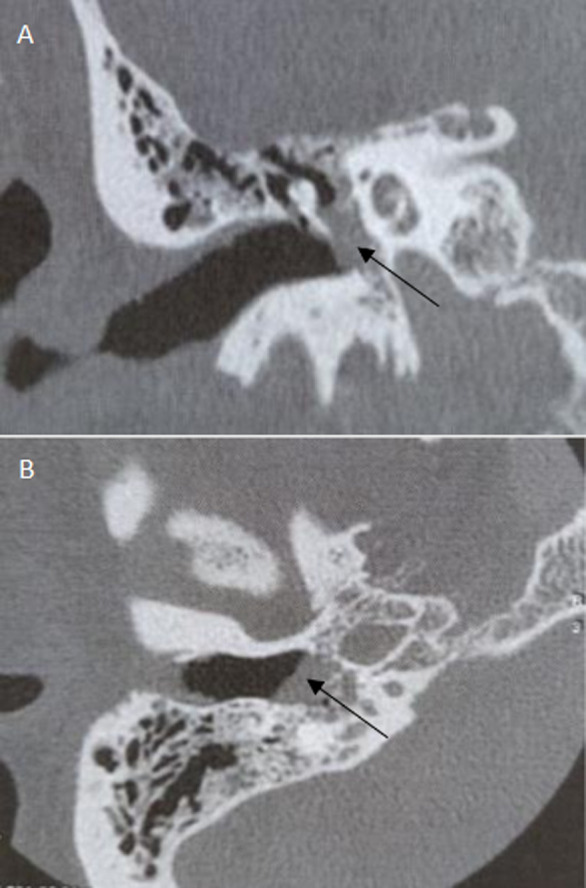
temporal bones computerized tomography (CT), coronal (A) and axial (B) sections, without contrast, showing a nonspecific, well-defined, 7 mm right nodular mass of soft tissue, arising from the hypotympanum and overlying the cochlear promontory
